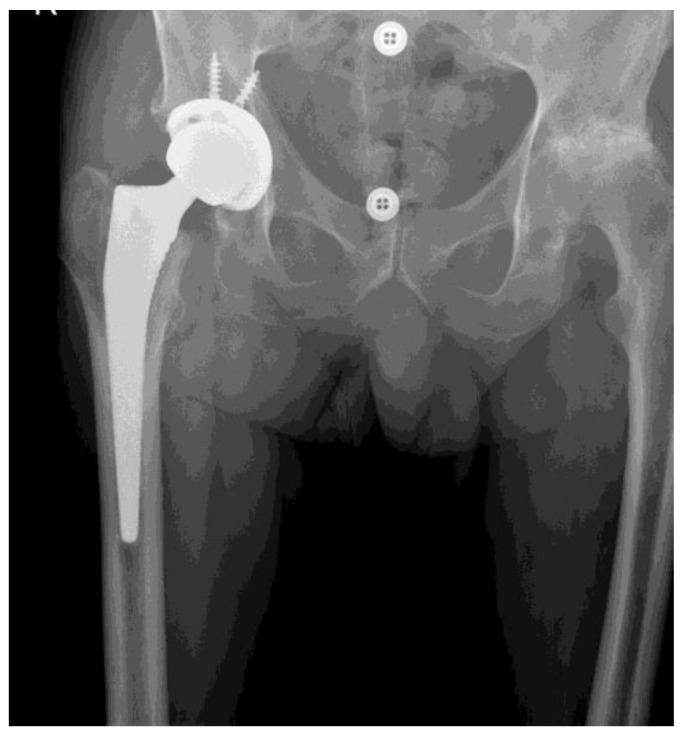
Pinnacle cup and Corail stem implants (DePuy Synthes, Warsaw, IN, USA) —radiograph after implantation—arthroplasty (from the archives of author A. Bohatyrewicz).
